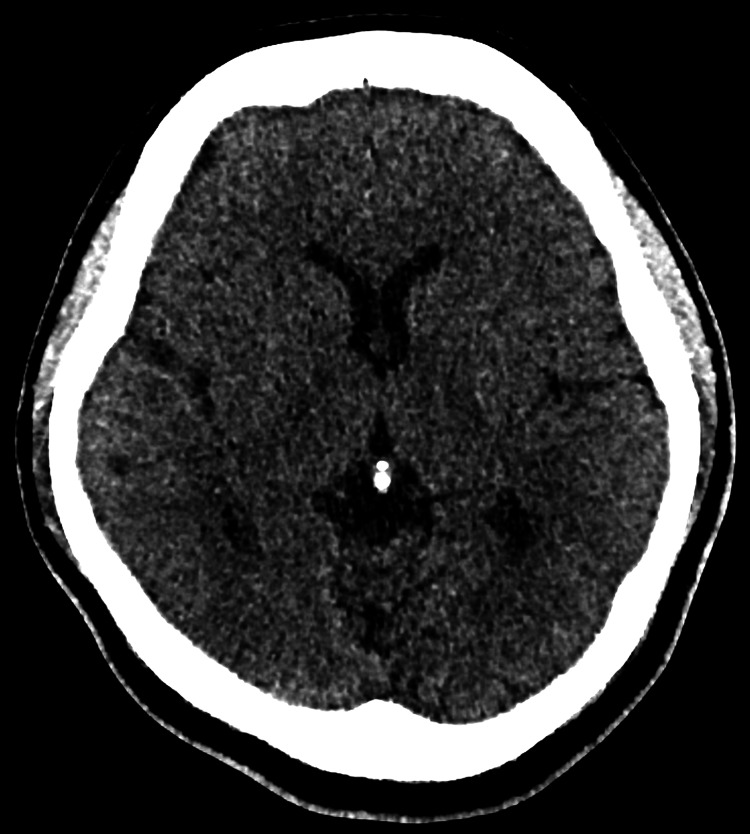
Non-contrast axial brain CT scan demonstrating no acute intracranial abnormalities
